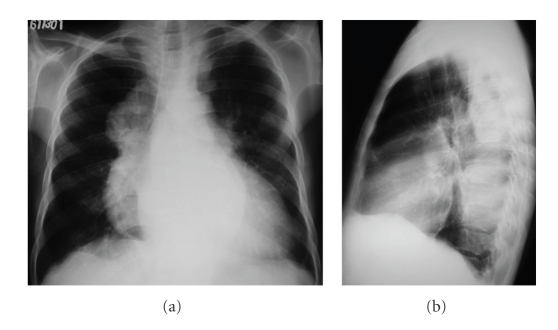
Anteroposterior (a) and lateral (b) chest radiographs showing large lobulated bilateral masses located in the retrocardiac region.
